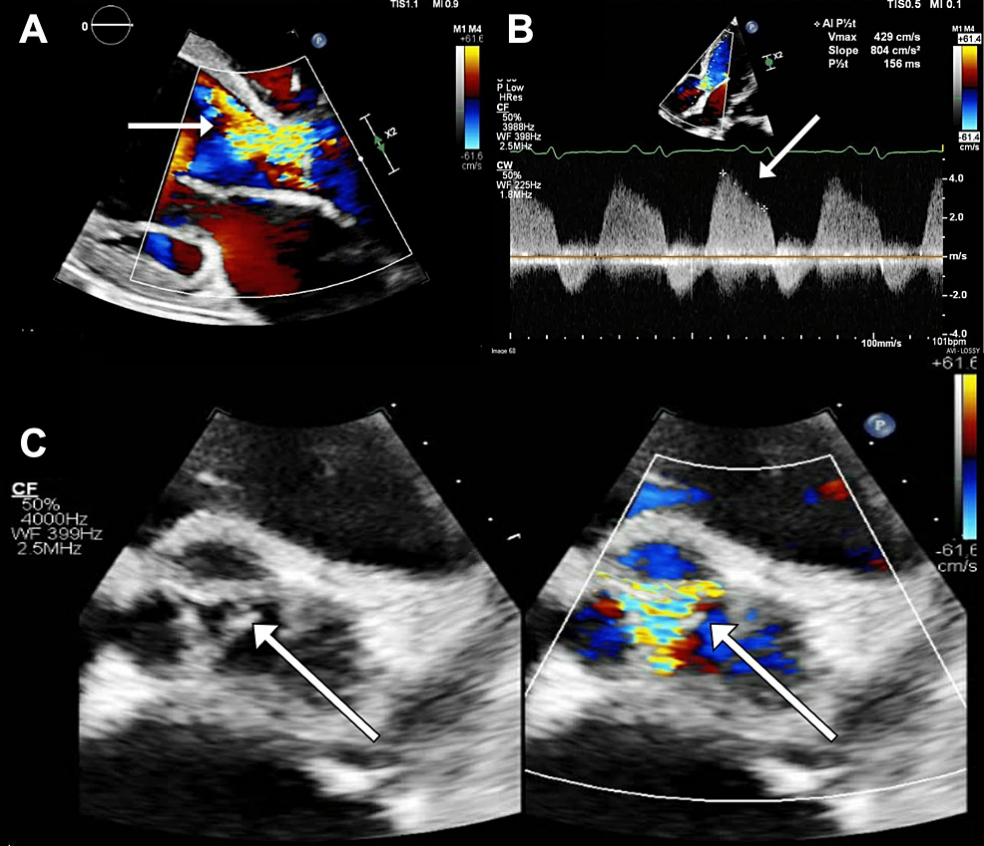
2D transthoracic echocardiography (A) 2D transthoracic echocardiography (TTE) parasternal long axis zoom in view with color Doppler of the aortic valve showing severe regurgitation. (B) 2D-TTE apical five-chamber zoom in view with color Doppler of the aortic valve showing severe regurgitation by continuous wave Doppler assessment (pressure half time of 156 milliseconds). (C) 2D-TTE short axis zoom in view of the aortic valve with and without color Doppler showing severe regurgitation during diastole.
